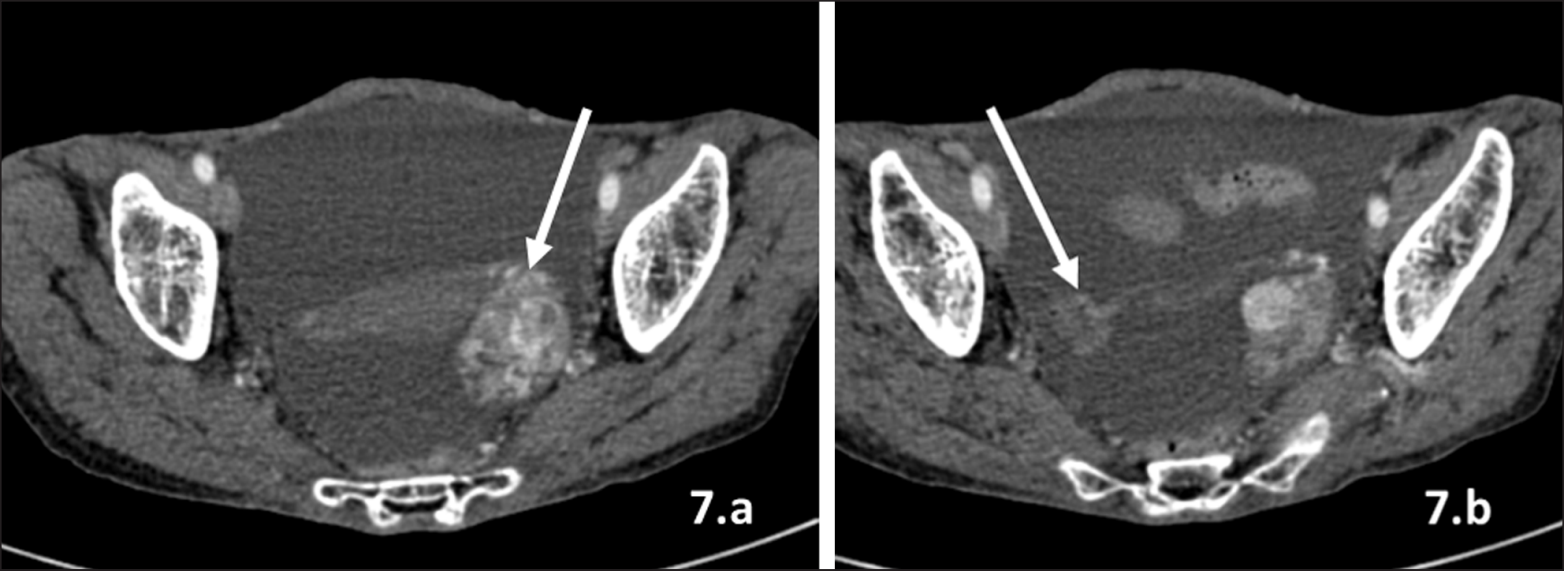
(a–b) Krukenberg tumour. Axial contrast-enhanced CT images of the abdomen in a post operative case of breast carcinoma reveal smooth-marginated solid cystic mass lesions in bilateral ovaries (white arrows) and ascites.
